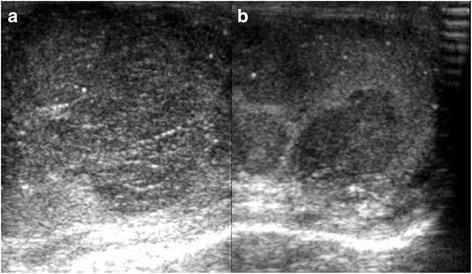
Ultrasonographic findings in patient 1. a Right testis. b Left testis
